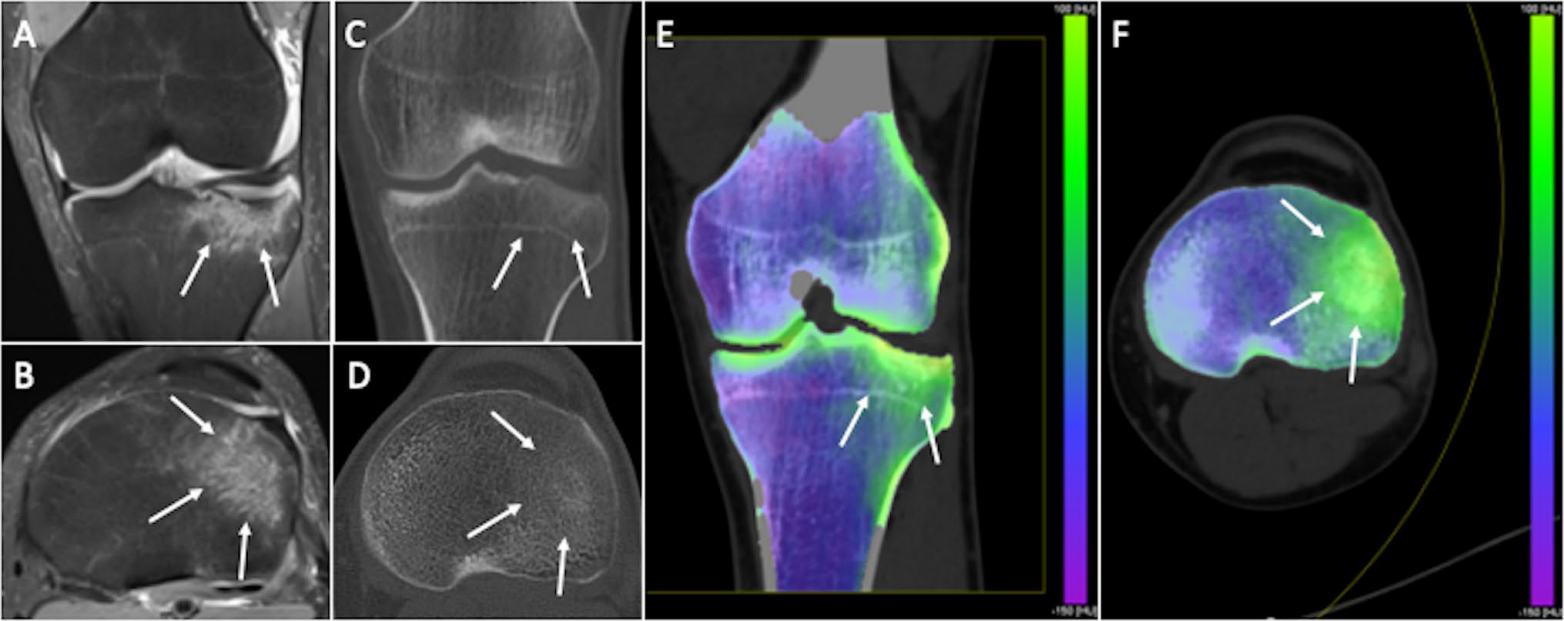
Traumatic tibial fracture in a 32-year-old woman. Coronal (a) and axial (b) fat-suppressed proton density weighted images show diffuse bone edema of lateral tibial plateau (arrows). Coronal reformat (c) and axial (d) standard CT images seem to show bone impaction of lateral tibial plateau as subtle subchondral hyperdensity (arrows), while the corresponding coronal (e) and axial (f) virtual noncalcium DECT images clearly demonstrate bone edema (arrows)
